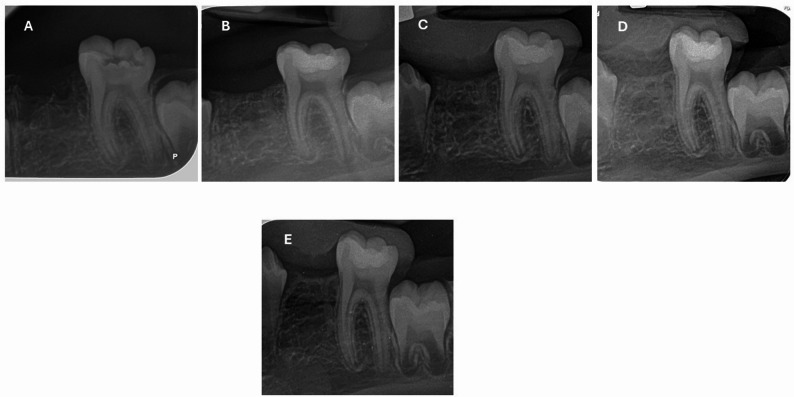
Radiographic follow-up of Powder/Liquid MTA; (A) preoperative, (B) immediate postoperative, (C) 3-month postoperative, (D) 6-month postoperative, and (E) 12-month postoperative
